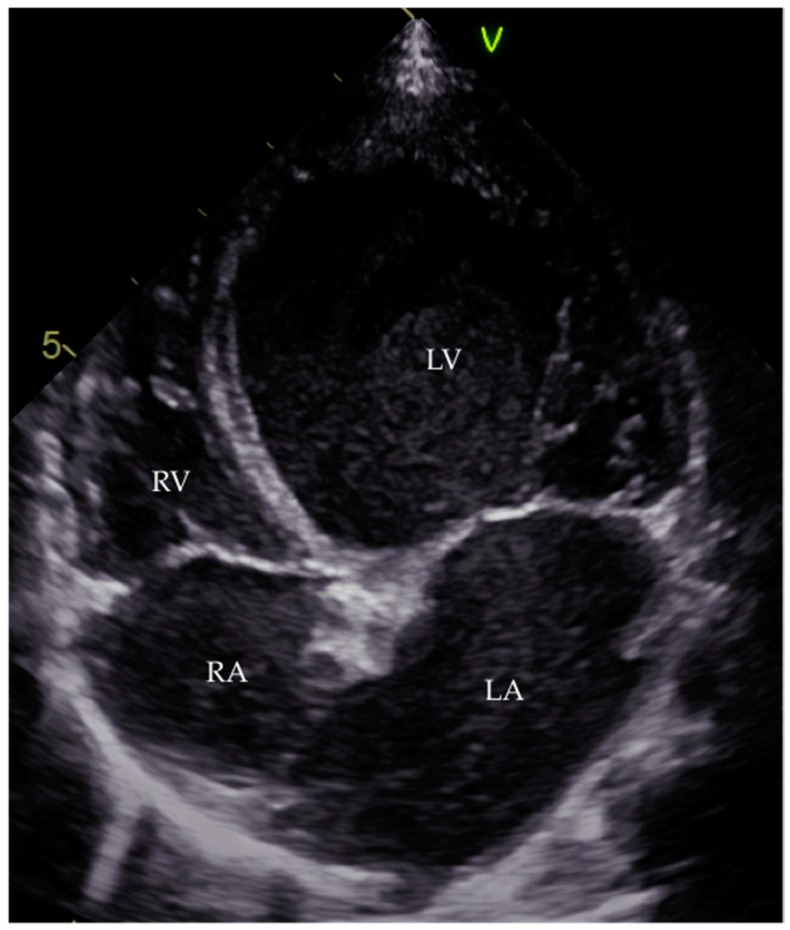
Four-chamber apical view echocardiogram of an 11-month-old boy with DCM. RA, right atrium; LA, left atrium; RV, right ventricle; LV, left ventricle.
